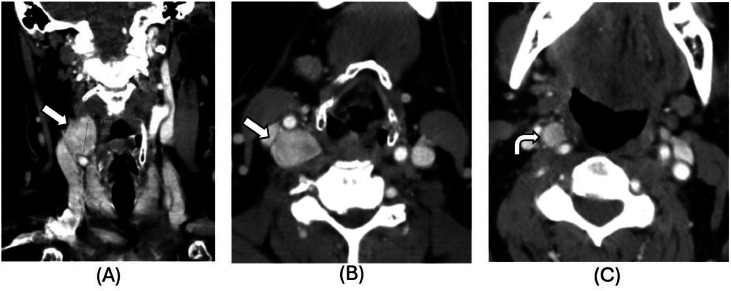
(A-C): Follow-up coronal (A) and axial (B, C) CT neck images demonstrating 2 distinct hyperdense masses, one inferior to the right carotid bifurcation near the posterior margin of the common carotid artery (white arrows, A-B) and the smaller lesion splaying the carotid bifurcation (curved arrow, C). Given the classical findings of carotid body tumor/paraganglioma, nuclear medicine Cu-64 Dotate PET/CT imaging was recommended, although metastatic adenopathy was still on the differential.
